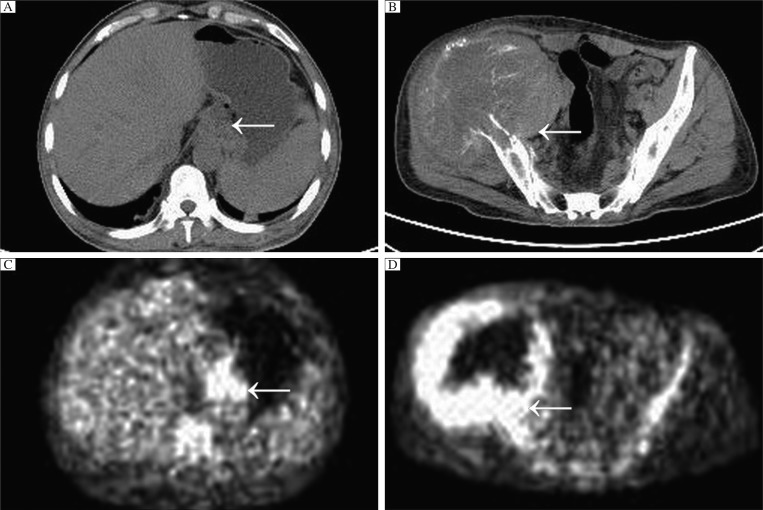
Pelvic metastasis of the cardia adenocarcinoma in a 49-year-old man. Axial unenhanced CT shows a thickened mass (arrow) in the cardia area (A) and an irregular-form soft tissue lump (arrow) in the right pelvis with osteolytic destructions of the right iliac bone (B). PET image exhibits high radioactive uptake (arrow) (C) and demonstrates ring-shaped high radioactive uptake (arrow) of the right pelvic mass (D).
